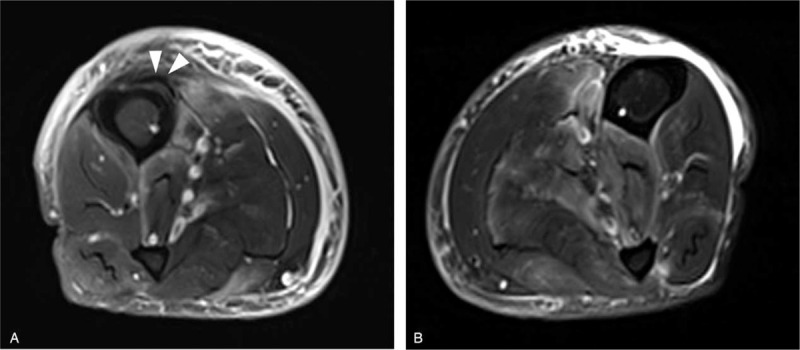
Axial fat-suppressed T2-weighted images of the right (A) and left (B) lower leg 5 months earlier. The right lower leg MRI shows periosteal elevation with mineralization at the tibial shaft (arrowheads). No periosteal reactions were noted in the left tibia or fibula. There are multifocal patchy edema in both lower leg muscles, especially prominent around both tibia, left fibula, and both intermuscular septa. Note diffuse swelling of the subcutaneous layers in both legs.
